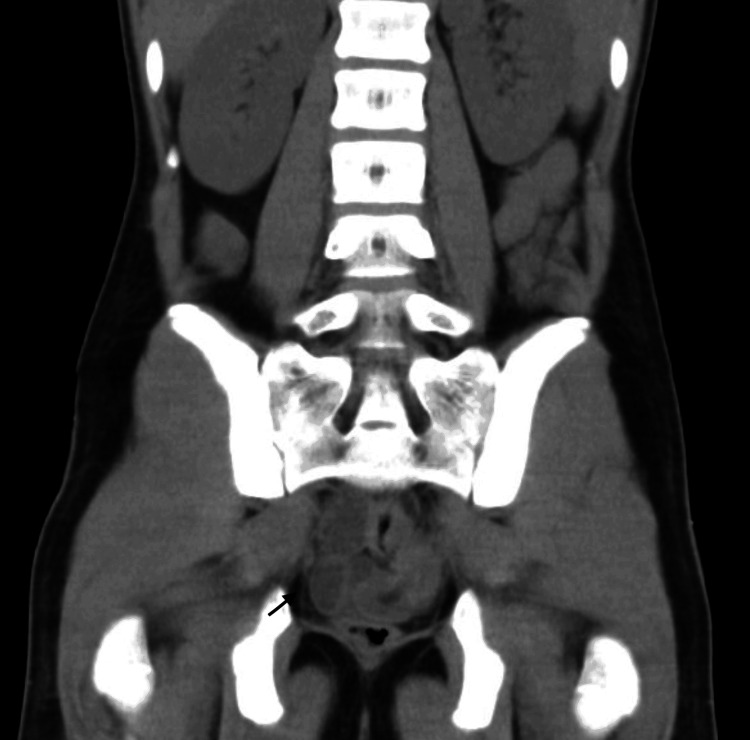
Coronal CT scan showing a longitudinal segment of intussusception with thickened walls and fatty content within the colon segment.
